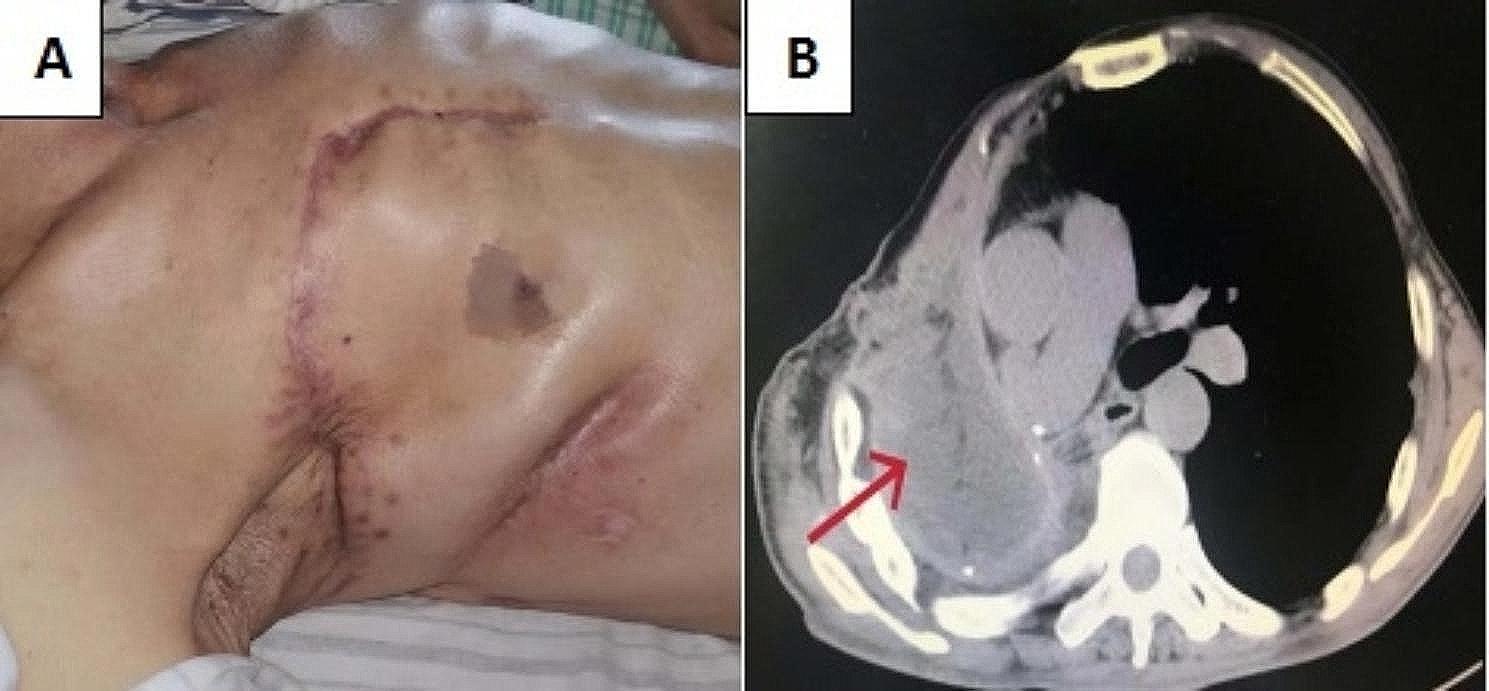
(A) Clinical data of postoperative patient. The postoperative chest incision recovered well. (B) Postoperative chest CT showed that the compound muscle flaps completely filled the abscess cavity, the empyema and chest wall sinus disappeared (red arrow)
